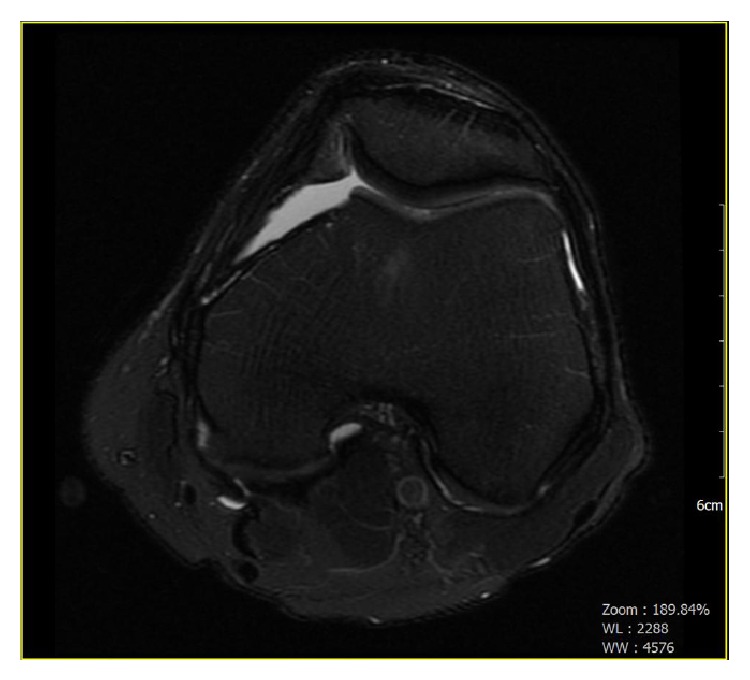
Nine-month postoperative radiograph. Unenhanced MRI of the left knee performed on a 1.5 Tesla magnet. Examination of the area of the femoral patellar joint where the patient is status-post a chondroplasty of the trochlear groove shows low T1 and high T2 signals in the subchondral bone. The articular surface of the chondroplasty defect shows a signal, which is isointense with articular cartilage. The thickness of the signal is less than that of the adjacent cartilage.
